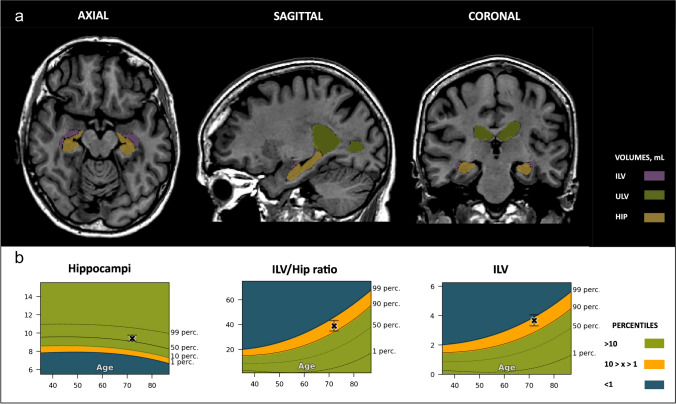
Subjective cognitive decline (SCD) case. Cross-sectional coronal T1-weighted image at the level of the medial temporal lobe and hippocampus with segmentation of the upper lateral ventricles (green), inferior lateral ventricle (purple), and hippocampus (yellow). A — Automated volumetric measurements *left*: axial view; *middle*: sagittal view; and *right*: coronal view. B — Population graphs *left*: hippocampal volumes of this subject showing the gray “x” marker in the green region just above the 50th percentile; *middle*: the ILV/Hip ratio of this subject showing the gray “x” marker in the orange region between the 90th and 99th percentiles. *Right*: ILV volumes of this subject showing the gray “x” marker in the orange region between the 90th and 99th percentiles. Color-coded population graph percentile interpretation; 1–90th percentile, green (normal); 90–99th percentile, orange (caution); > 99th percentile, dark blue (abnormality)
